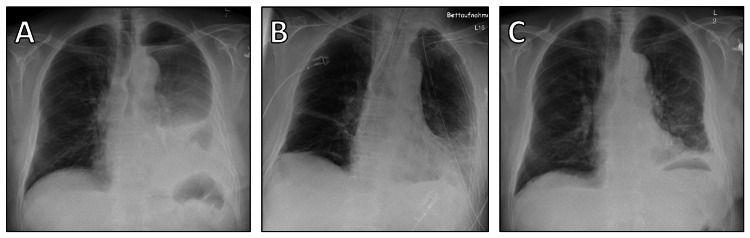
Chest X-rays at different stages of treatment. (A) Preoperative image displaying the recurrent tumor's impact on lung fields and mediastinal structures. (B) Immediate postoperative image indicating the successful resection and the initial postoperative state of the thoracic cavity. (C) Image at discharge showing the recovery progress and the state of the thoracic cavity post-treatment.
